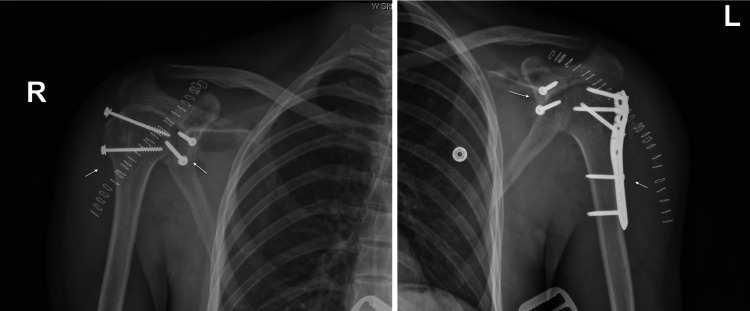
Bilateral shoulder x-rays taken after open reduction of the fractures and internal fixation and Latarjet
